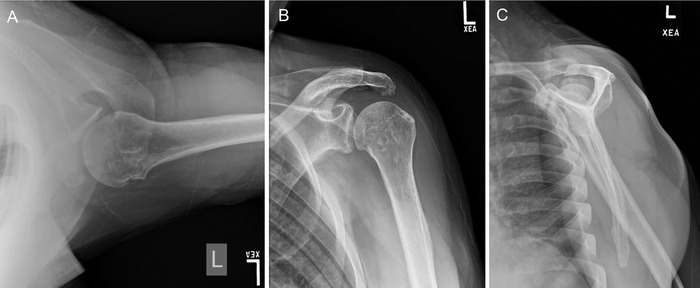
Preoperative radiographs in (A) axillary view, (B) anterior-posterior Grashey view, and (C) scapular Y view show cystic changes of the greater and lesser tuberosities and subacromial enthesopathic spurring consistent with rotator cuff pathology.
